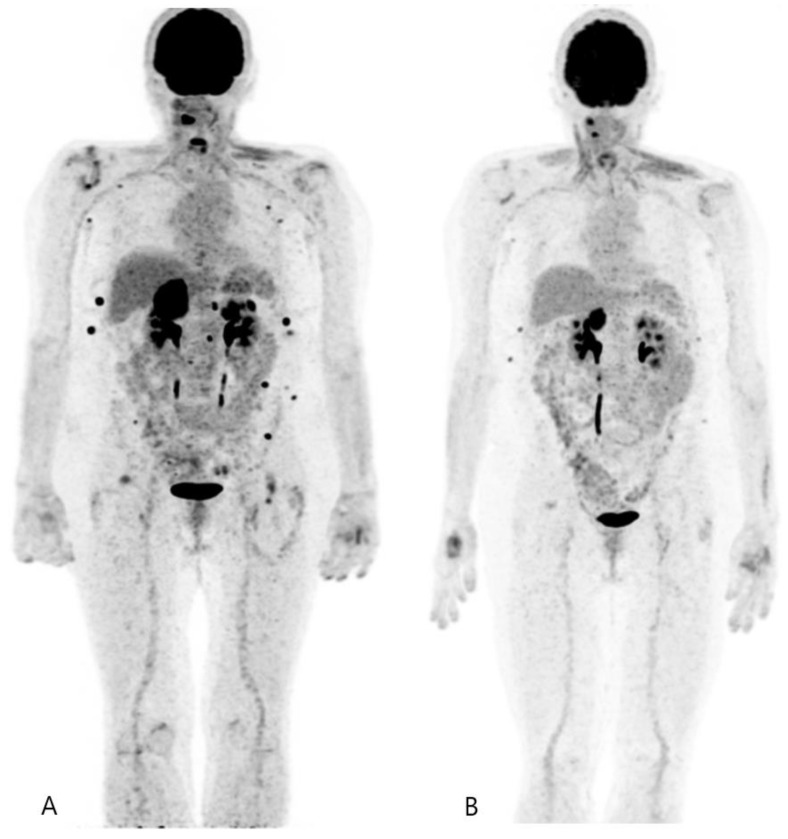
FDG PET/CT scans evaluating the response of multi-metastatic iodine-refractory papillary thyroid carcinoma following treatment with tyrosine kinase inhibitors. (A) MIP image obtained before treatment. (B) MIP image three months post-treatment with tyrosine kinase inhibitors, showing a partial response.
